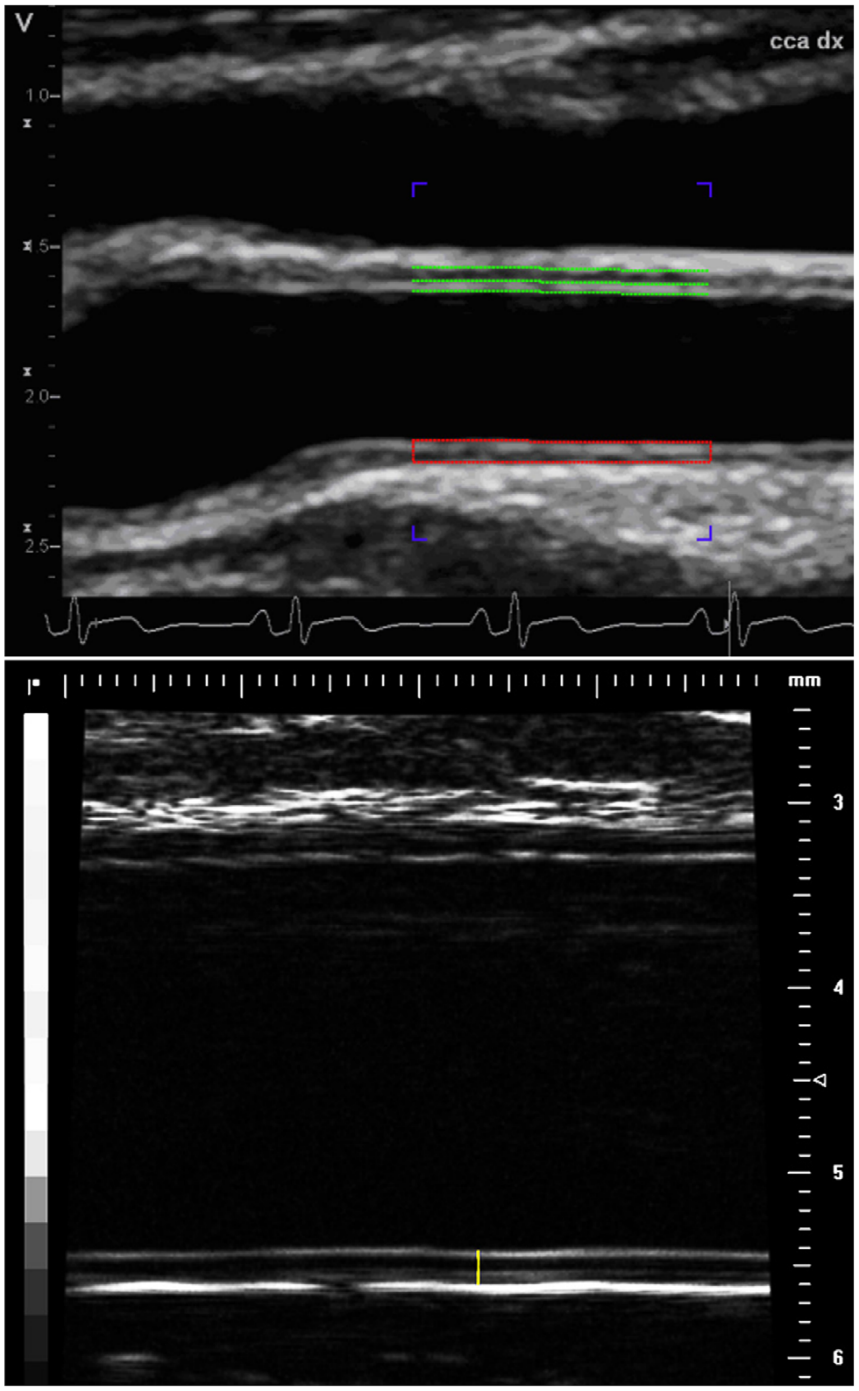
Carotid and radial artery ultrasound images. The red rectangular outline in the upper panel delineates the measurement of the right common carotid artery far wall intima media thickness (IMT_cca_) and grayscale median (IM-GSM). The vertical yellow line in the lower panel indicates the intima media thickness of the radial artery (IMT_rad_).
